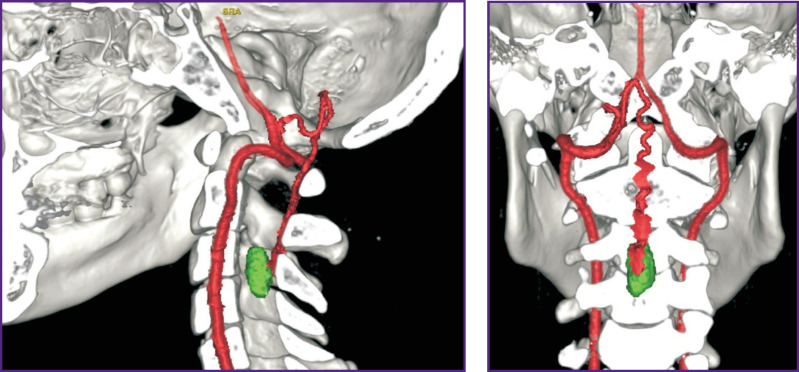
3D reconstruction based on CTA of intramedullary hemangioblastoma vascular anatomy at С_3_–С_4_ level (source: authors)
